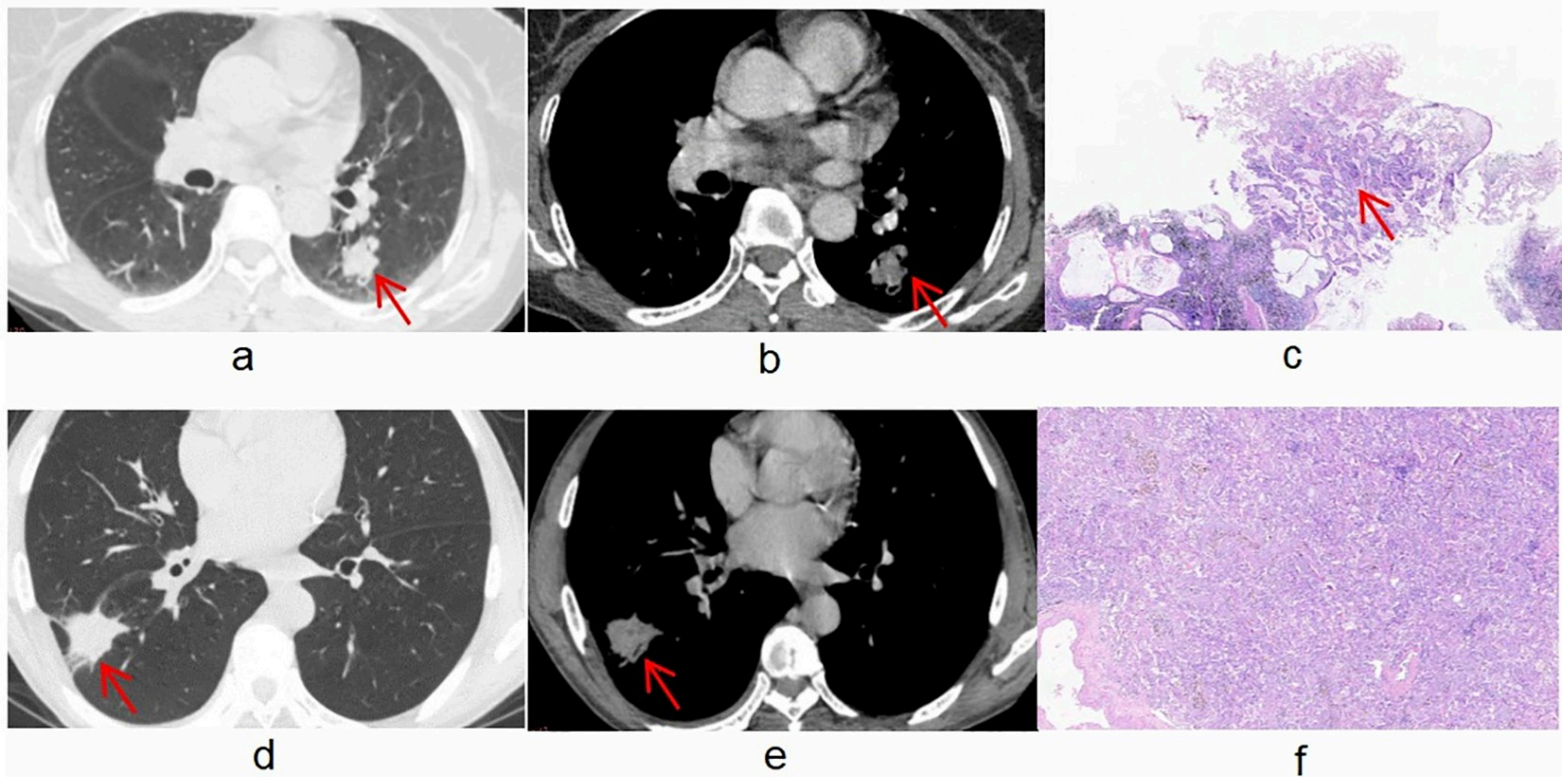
Chest CT and pathological diagnosis of the pulmonary larger solid nodules and masses (a, b, c, d, e, and f). Lesions are shown by arrows. Chest CT images (a and b) of a 61-year-old female patient revealed a solid nodule in the lower lobe of the left lung, and this nodule was pathologically (c) diagnosed as pulmonary adenocarcinoma. Chest CT images (d and e) of a 60-year-old male patient revealed a solid mass in the lower lobe of the right lung, and this mass was pathologically (f) diagnosed as organizing pneumonia.
